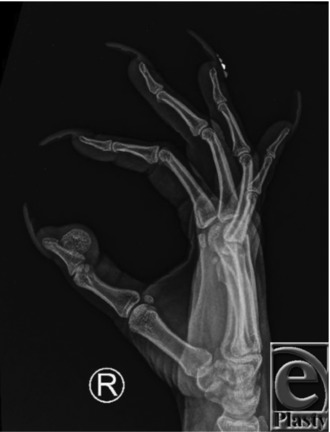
Preoperative x-ray showing pedunculated mass off volar proximal aspect of distal phalanx.
